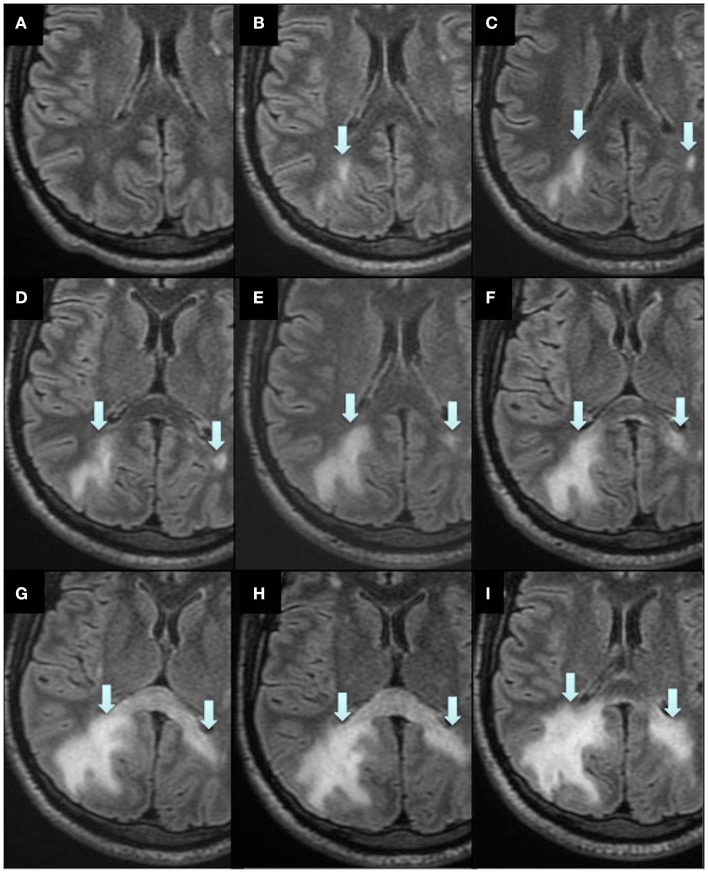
Magnetic resonance imaging scans during the course of PML and IRIS. Axial reconstructions from 3D Flair acquisition. Routine MRI 1 year before PML diagnosis (A), when PML was diagnosed (B) and subsequent scans directly after plasma exchange (C), 1 week (D), 2 weeks (E), 4 weeks (F), 5 weeks (G), 1.5 months (H), and 2.5 months (I) after plasma exchange. Arrows indicate the PML lesions.
